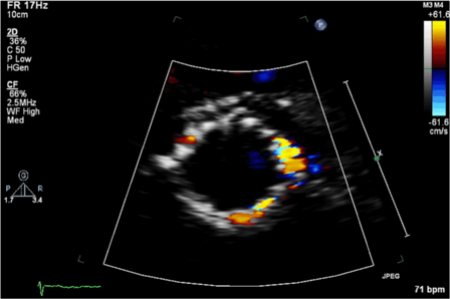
Multiple PVL jets seen at 2–3 o’clock, 4–6 o’clock, and 10 o’clock in the parasternal short-axis
